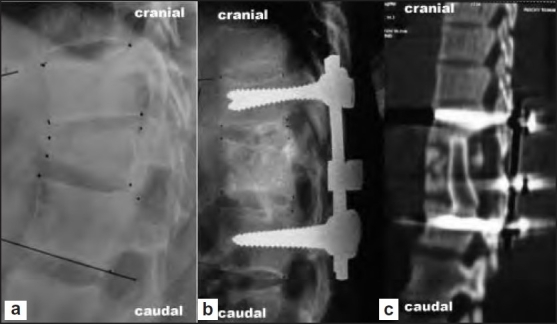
(a) Preoperative X-ray of dorsolumbar spine lateral view of 32 years old male with burst fracture (type A) of D12 with preoperative kyphosis of 32°. (b) Immediate postoperative X-ray lateral view after single-stage anterior decompression, bone grating, and pedicle screw fixation via extrapleural retroperitoneal approach, showing correction of kyphosis and well-placed bone graft. (c) Six months postoperative migsaggital reconstructed CT image showing graft incorporation and final kyphosis of 11°
